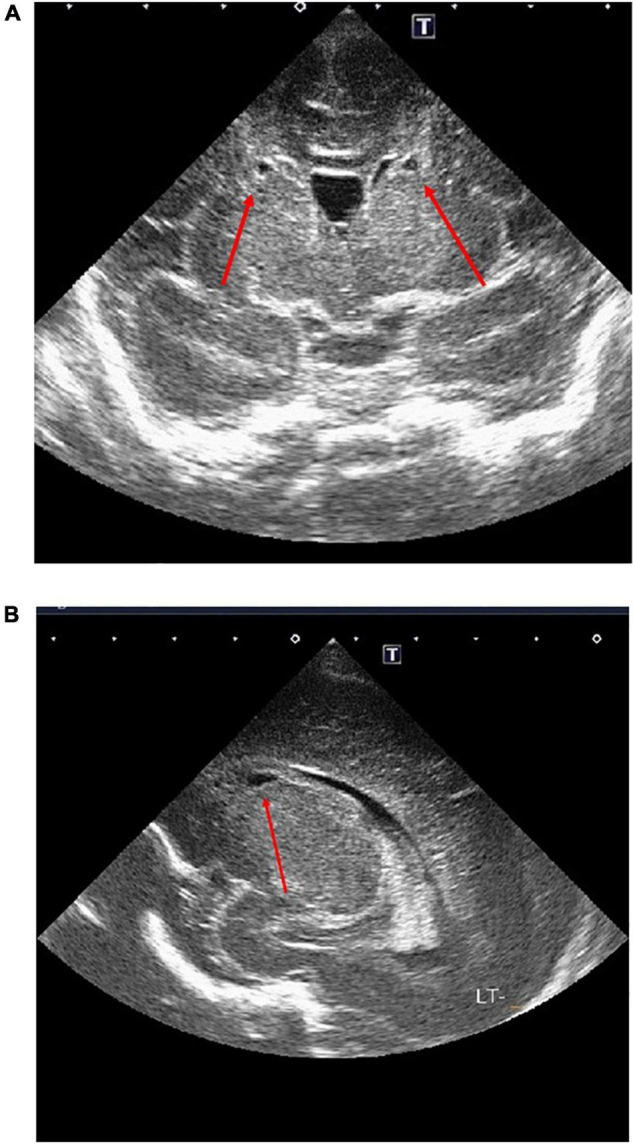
(A,B) Head ultrasound. Day 0 Red allows show bilateral cystic lesions by the anterior horn of the each ventricles.
